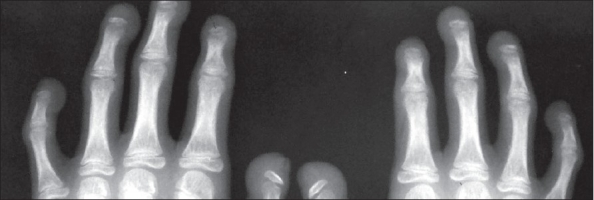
Radiograph of both hands shows acro-osteolysis
